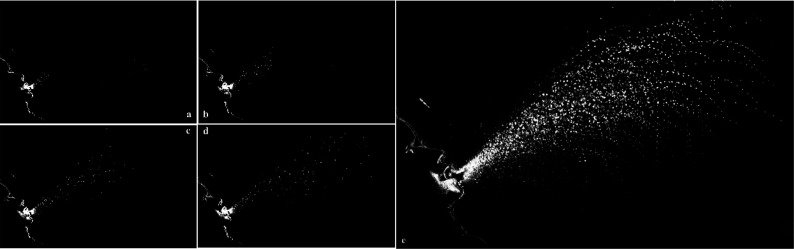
The development of fluid droplets. The image sequence shows multiple snapshots of ultrasonic scaling in the planar slice $Z/D_{L}=0$ (central axis) for the moment (a) 0.02, (b) 0.05, (c) 0.09, and (d) 0.24 s. Figure 5(e) the visualization of expelled fluid droplets and their trajectories. The images are taken from video 1. Multimedia view: https://doi.org/10.1063/5.0048848.110.1063/5.0048848.1
